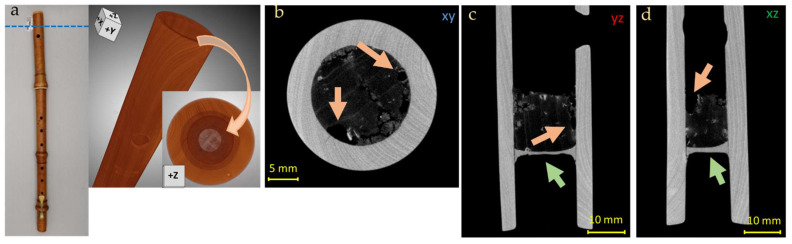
Original and 3D render (a) and CT horizontal (b), lateral (c) and vertical (d), slices of the Transverse Flute head, in which some voids in the cork (orange arrows) and the wax layer placed on (green arrow) are observed. Blue line in (a) indicates the height of the flute head in which the cork is inserted.
